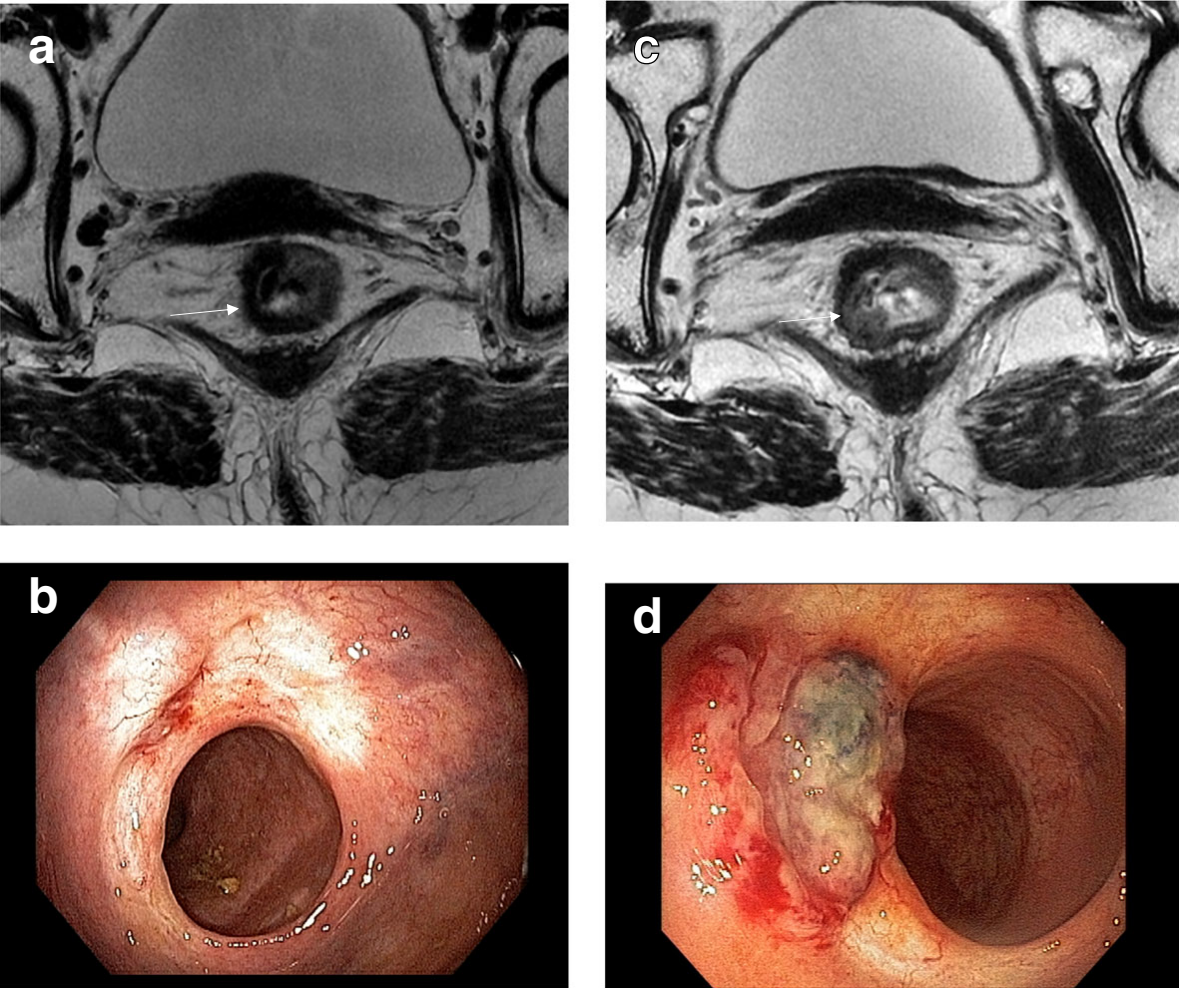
Luminal regrowth detected by MRI and endoscopy. a High-resolution T2WI restaging after 8 weeks of completion of CRT shows a low signal intensity fibrotic full-thickness scar extending from 5 to 12 o’clock (arrow). The patient was referred to watch-and-wait. b The endoscopic view of the treated lesion after 8 weeks of completing CRT shows whitening of the mucosa with some telangiectasia and no significant residual ulceration. c Follow-up after 16 months of completion of CRT shows increased signal intensity where the scar was previously seen from 6 to 9 o’clock (arrow). The patient was referred to salvage resection with total mesorectal excision. A clear margin resection was performed. d The endoscopic view of the treated lesion after 16 months of completing CRT shows evident tumor regrowth
